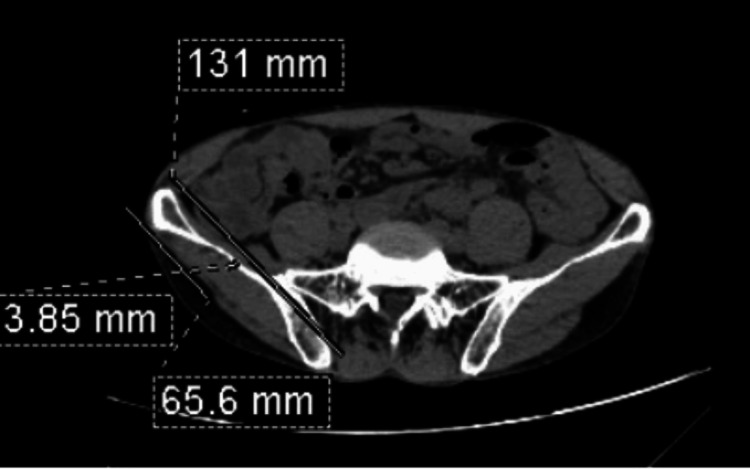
Axial CT section of the abdomen shows the measurement of the iliac bone thickness. There is cortical thinning of the iliac bone with fibrous marrow.
